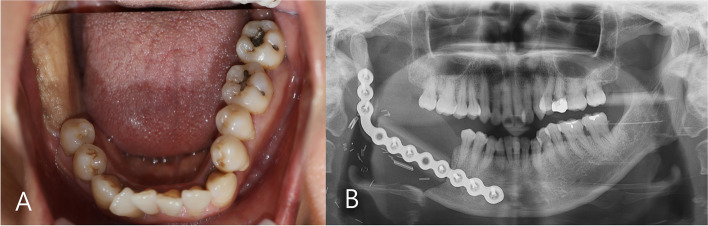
Postoperative 4-month follow-up. A Intraoral skin paddle maintained in good condition. B Panoramic radiography view indicated favorable healing. Fibular bone had satisfactorily adjusted with residual mandible and restored well
